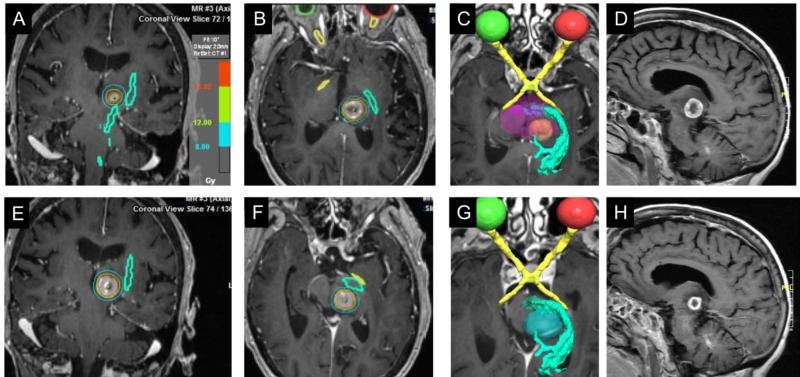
SRS treatment planning using DTI-MRI images to localize corticospinal tracts For SRS treatment planning, an MRI with DTI was used to establish the proximity of the target lesion to the CST and calculate dose-volume histograms for the CST. Coronal (A,E) and axial (B,F) images from the SRS treatment plan showing MRI-DTI defined CST (green), gross tumor volume (GTV; orange), and isodose lines (blue - 8Gy; green - 12 Gy; red - 14Gy). (C,G) Axial 3D renderings of CTS (bright green) relative to the GTV (C) and 8 Gy isodose volume (G). The optic pathway is outlined (yellow; C,D) as well as the brainstem (purple; C). Pretreatment (D) and one-month post-treatment (H) MRI with gadolinium show a marked reduction in tumor volume. DTI: diffusion tensor imaging; CST: corticospinal tract
